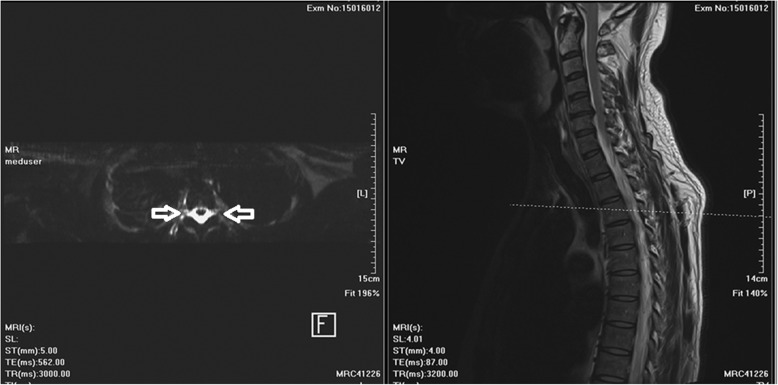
T2-weighed MR image reveals contrast enhancement along the bilateral neural sleeves of T2-T3. The lesion reveals a high signal intensity stripe with the length less than the width of the thecal sac. According to the typing system proposed by Chen et al. [4], a Type C lesion was defined at T2-T3. Arrow: CSF leak lesions
